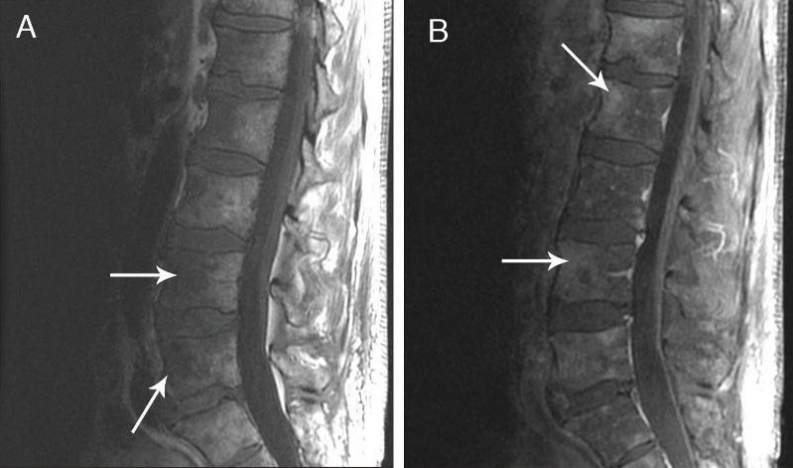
MRI of spine demonstrating multifocal hypointensities (arrows) sparing the intervertebral disks in T1-weighted images (a). The same lesions (arrows) appear hyperintense on T2-weighted images (b). No inflammation of the paraspinal muscles or abscess was identified.
